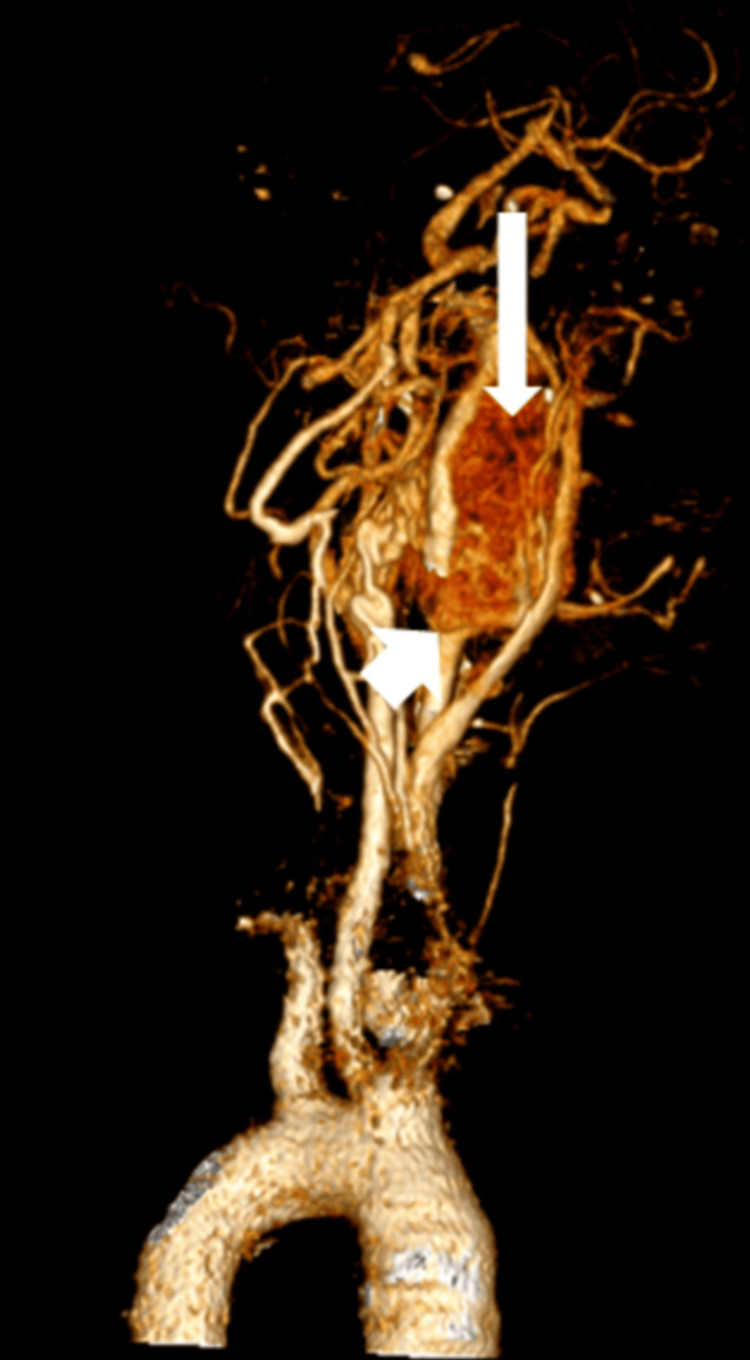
Volume-rendered 3D reconstruction of CECT neck Volume-rendered 3D reconstruction of CECT neck showing mass lesion (long white arrow) causing splaying of ECA and ICA (short white arrow). CECT: contrast-enhanced computed tomography; ECA: external carotid artery; ICA: internal carotid artery
